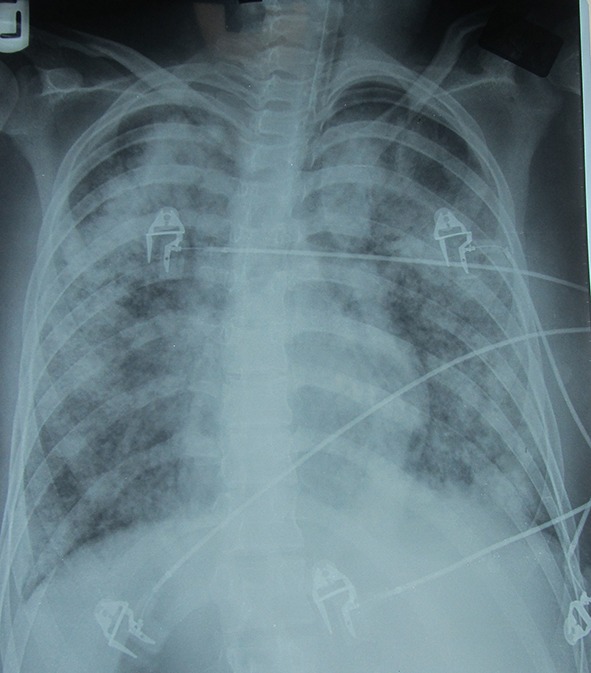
Chest X-ray AP: Bilateral diffuse alveolar infiltration.
